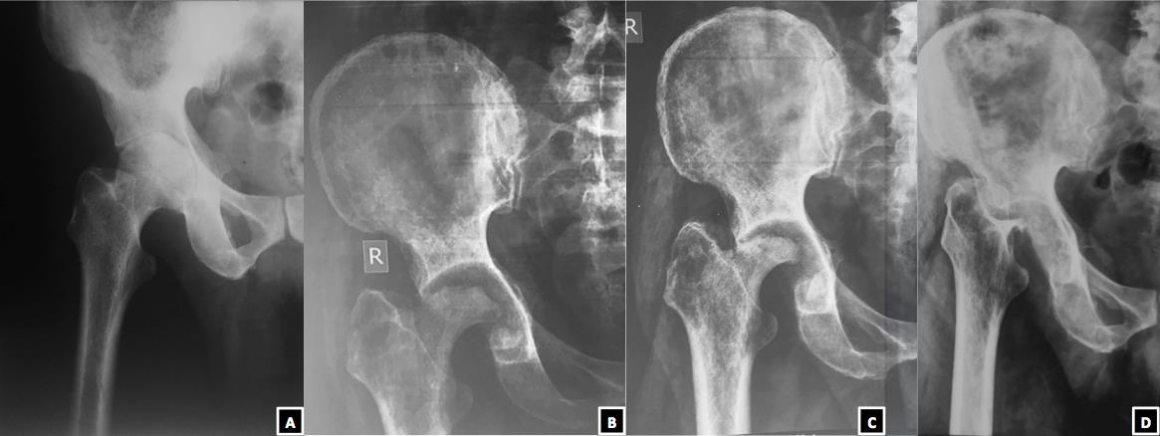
Serial x-rays of the right hip of the patient in Anteroposterior view showing complete resolution of the femoral head at the latest follow up
